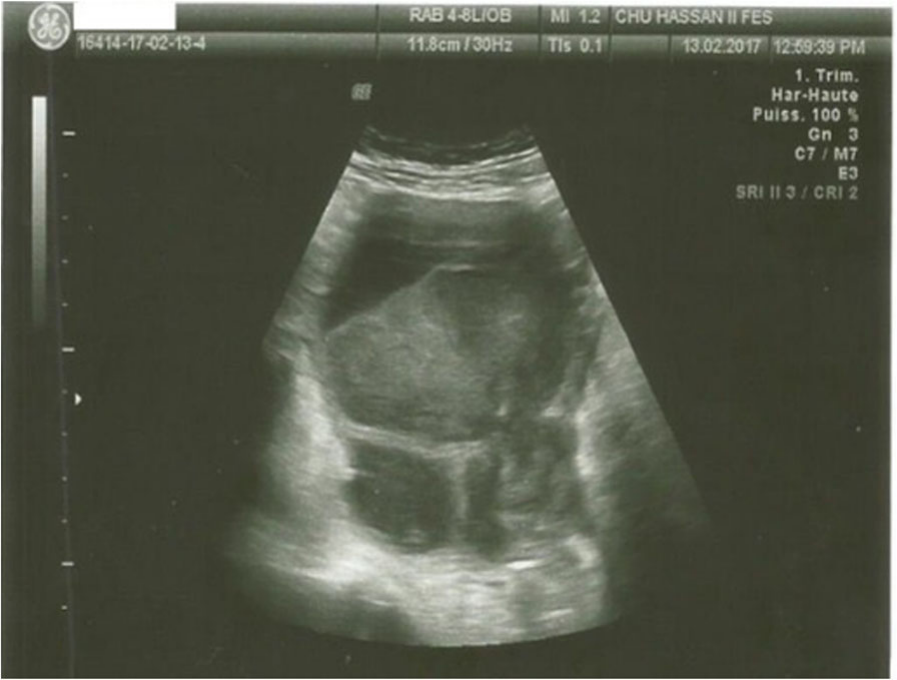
Pelvic ultrasound showing changes in cysts with a thickened wall and heterogeneous content with the appearance of a fluid level
